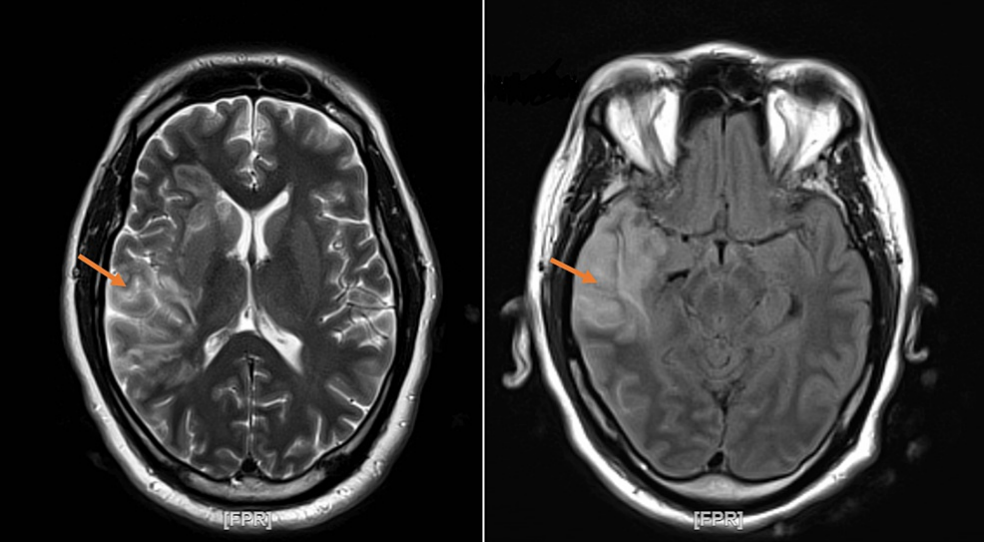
MRI of the brain Axial FLAIR (left) and axial T2-weighted image (right): orange arrow shows hemorrhagic conversion of the right MCA stroke. MRI: magnetic resonance imaging, FLAIR: fluid-attenuated inversion recovery, MCA: middle cerebral artery
